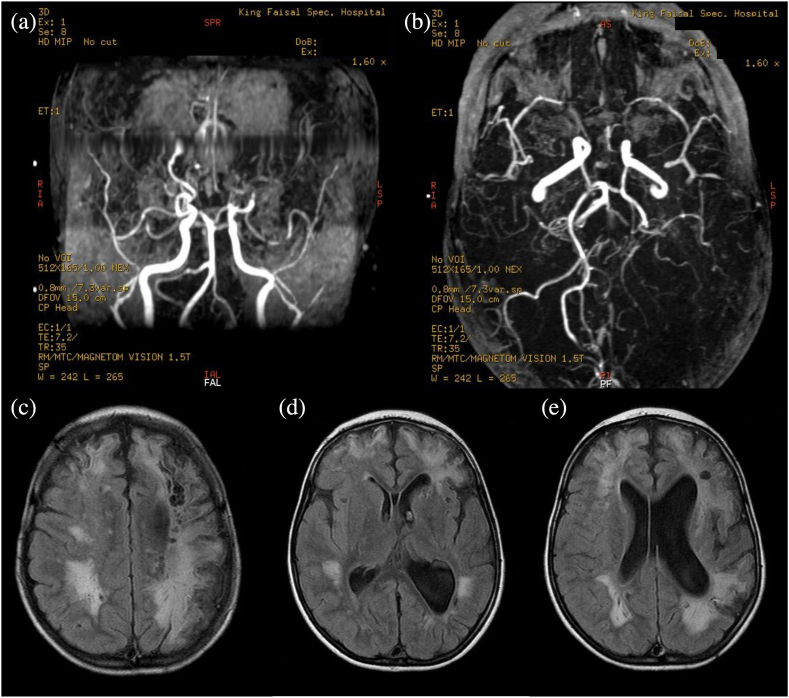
The MRA of the intracranial circulation on (a) coronal, and (b) axial view, showing significant bilateral supraclinoid ICA loss of normal flow, with poor flow in the middle cerebral arteries and the anterior cerebral arteries bilaterally. Prominent collateral flow is also detected in the perforator branches. These findings are consistent with a moyamoya syndrome pattern. Posterior circulation shows good flow in the basilar artery as well as in the posterior cerebral arteries on both sides. FLAIR MRI taken (c) below the level of the caudate nucleus, (d) at the level of the caudate nucleus and genu of the corpus collosum, and (e) at the level of the occipital horns of the lateral ventricles, showing multiple infractions in both hemispheres. Significant cystic encephalomalacic changes are seen involving the supratentorial brain bilaterally, with more involvement of the left hemisphere. The frontal and parietal areas are also involved. Periventricular hyperintensity is detected, especially in the parietal regions on both sides, most likely representative of small vessel ischemic changes (however, associated white matter disease cannot be completely excluded). There is significant prominence in the perivascular spaces, with multiple well-defined lacunar infarcts in the basal ganglia, especially in the left caudate head. Ex vacuo enlargement of the left lateral ventricle is present, related to the diffuse ischemic changes and volume loss. Thinning of the corpus callosum is present related to the overall brain atrophy and ischemic changes. These findings are most likely secondary to vascular events, especially with the significant vascular abnormalities consistent with moyamoya type pattern.
